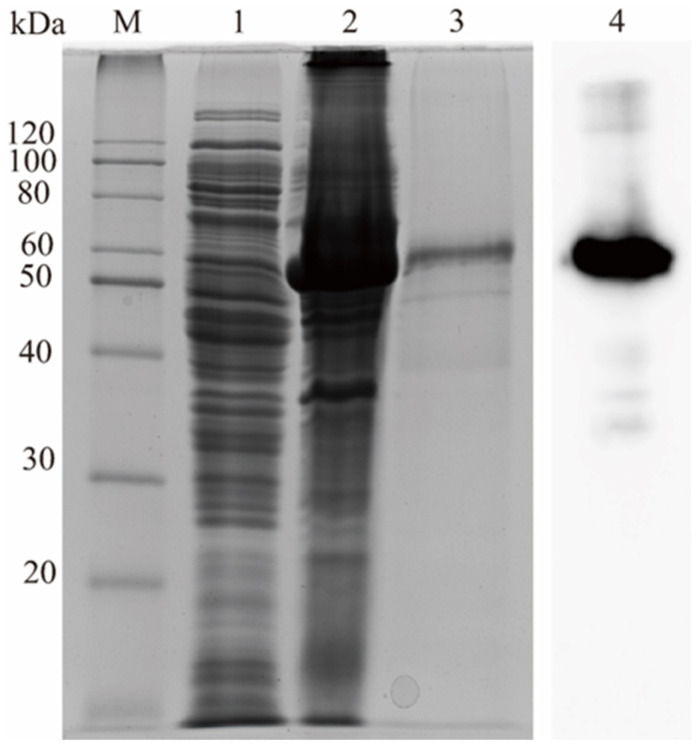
Analysis of RuXyn854 using SDS-PAGE and Western blotting. M, protein marker; 1, non-transformed *E. coli* BL21(DE3); 2, RuXyn854 transformants induced with 0.4 mM IPTG; 3, purified RuXyn854; 4, Western blot analysis of supernatant from the ultrasonication of RuXyn854 transformants.
